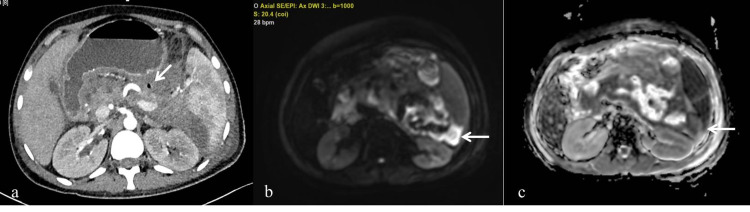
A 26-year-old-male, presented with alcohol-induced acute pancreatitis, fine-needle aspiration analysis yield infective collection with Escherichia coli growth on microbial culture. (a) Axial contrast CT pancreatic phase showing pancreatic parenchymal destruction with collection in the lesser sac, anterior pararenal space and air foci within (arrow). (b, c) Diffusion-weighted MR image at b-value of 1,000 s/mm^2^ and corresponding ADC map showing diffusion restriction in the collection (arrow).
